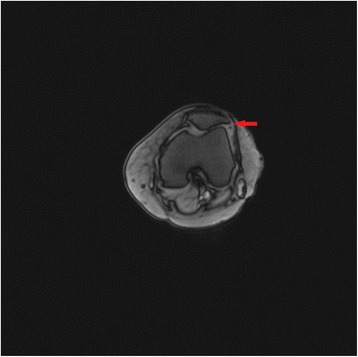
MRI features of the patients before surgery
